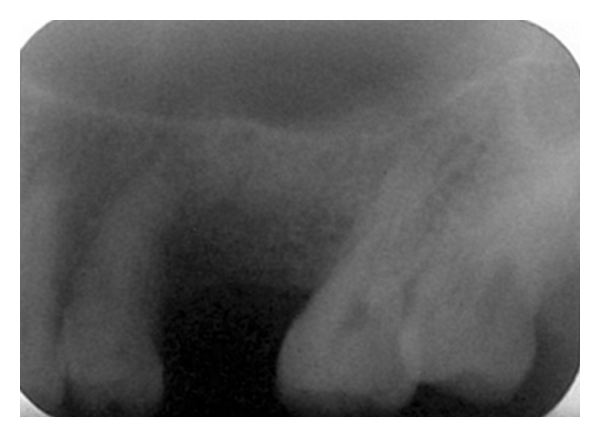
IOPA radiograph of maxillary right posterior region showing a partial radiolucency superimposed on the edentulous region.
